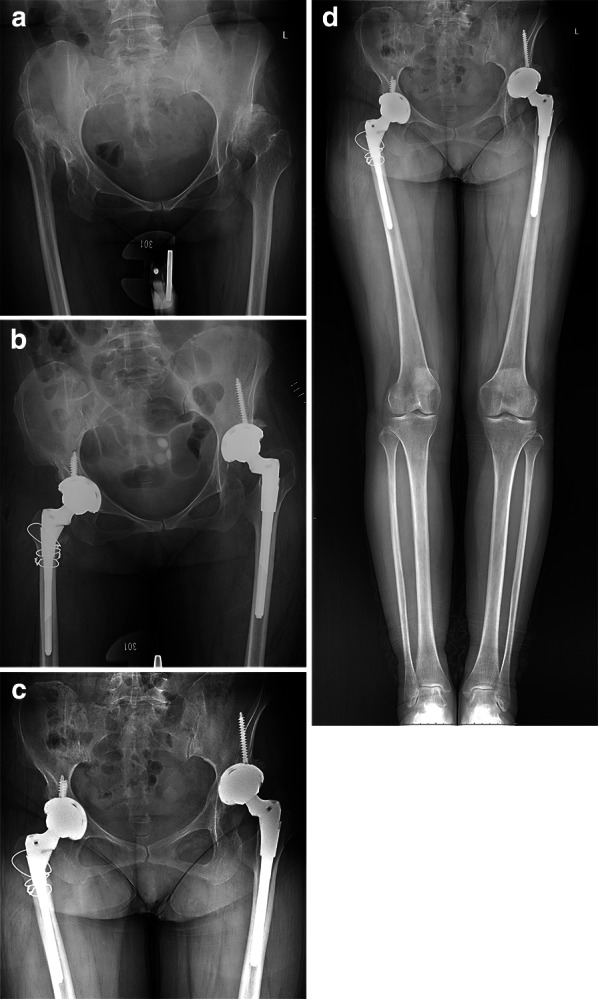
Preoperative anterior–posterior X-ray highlighting a bilateral DDH (right hip as Crowe IV and left hip as Crowe III) in a 49-year-old female patient (a). Postoperative X-ray showing an asymmetrical reconstruction of the Crowe III hip with high hip center and the Crowe IV hip at the anatomical position (b). SSTO was performed in the Crowe IV hip, and the osteotomy length was 3.5 cm. At final follow-up, radiographic evaluation after 3.5 years showing no loosening (c). Full-length standing anteroposterior radiograph showing that both lower limbs were of equal length (d)
